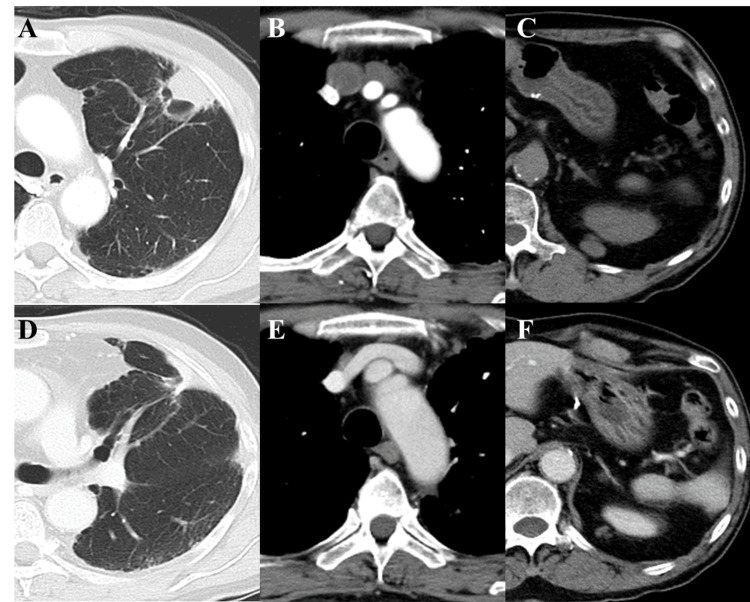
Treatment response Comparison of CT images before (A-C) and after (D-F) treatment. Lesions have improved significantly.
